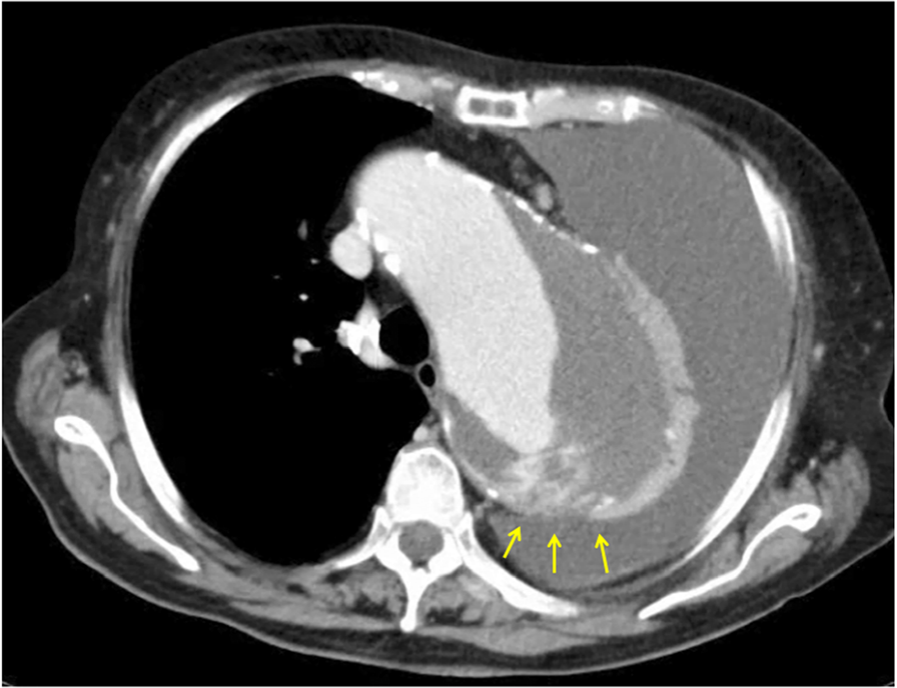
Computed tomography with contrast enhancement in a patient with aortic rupture. Arrows indicate the rupture site
